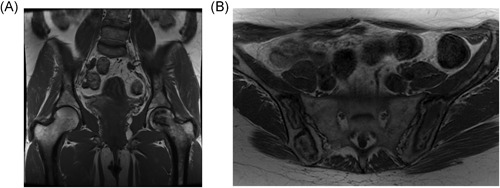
Osteonecrosis. (*A*) Typical. T1‐weighted MR image of the pelvis, showing a geographic area of low signal in the head of the left femur. Note that the joint surface remains intact in this case; therefore, there is no deformity or degenerative change in the hip joint. (*B*) Atypical. T1‐weighted MR image of the pelvis in a different patient showing diffuse low geographic area of low and high signal through the pelvic bones bilaterally. The radiologic changes in (*B*) occurred gradually over years, on enzyme therapy, and without the typical symptoms of bone crisis
